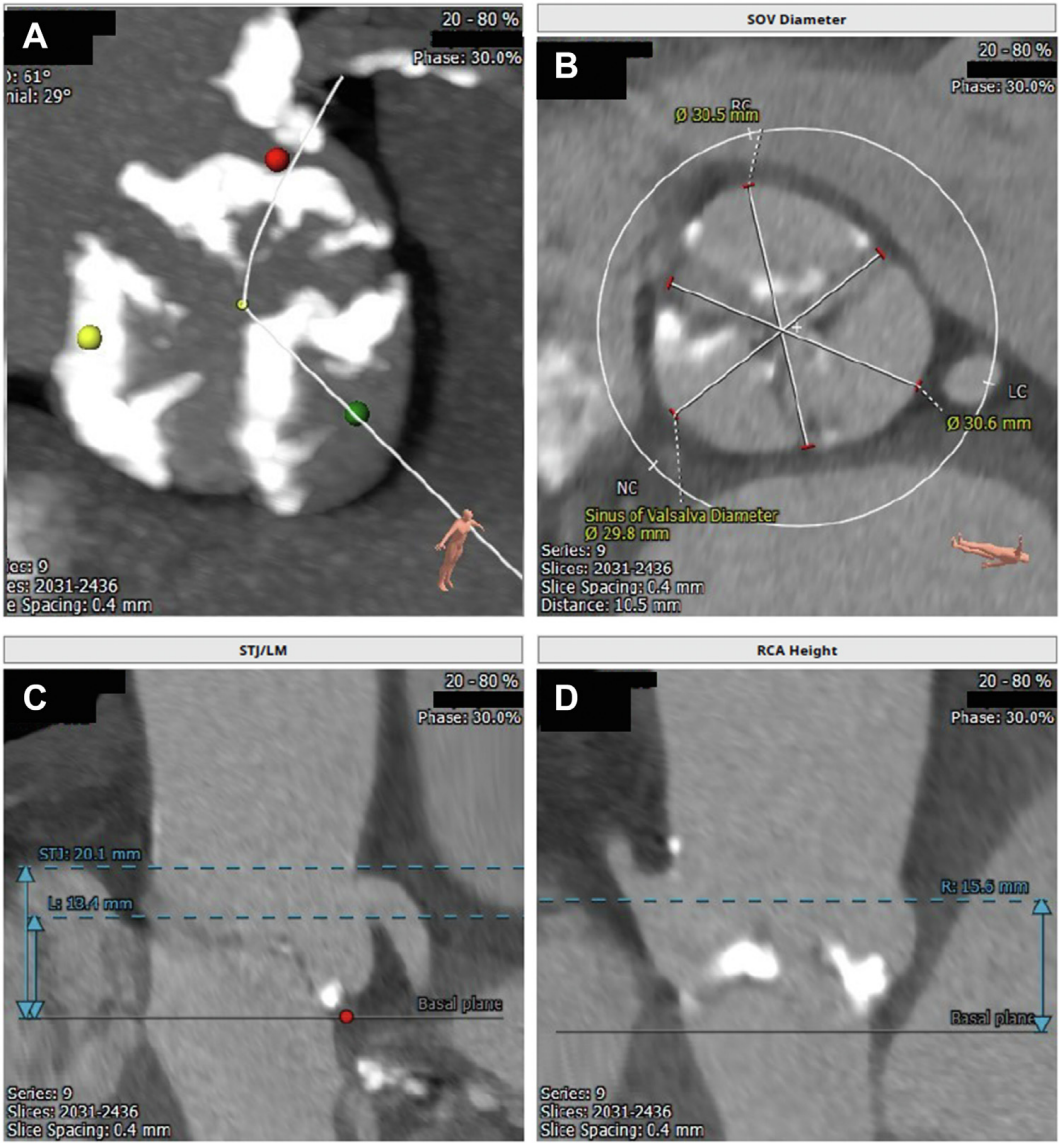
Calcified Transcatheter Aortic Valve The transcatheter aortic valve replacement protocol computed tomography showed a moderately calcified aortic valve and minimal ascending aortic calcification. LM = left main coronary artery; RCA = right coronary artery; SOV = sinus of Valsalva; STJ = sinotubular junction.
